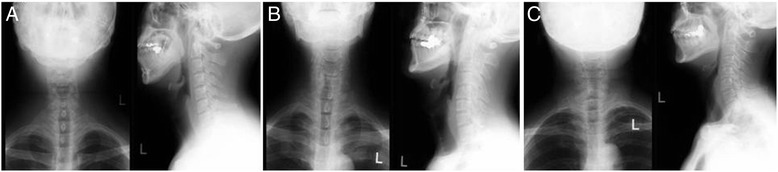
X-ray images of the larynx a few months after surgery. No subglottic stenosis was observed in any of the cases. a X-ray image of Case 1 at 5 months after surgery. b X-ray image of Case 2 at 4 months after surgery. c X-ray image of Case 3 at 7 months after surgery
